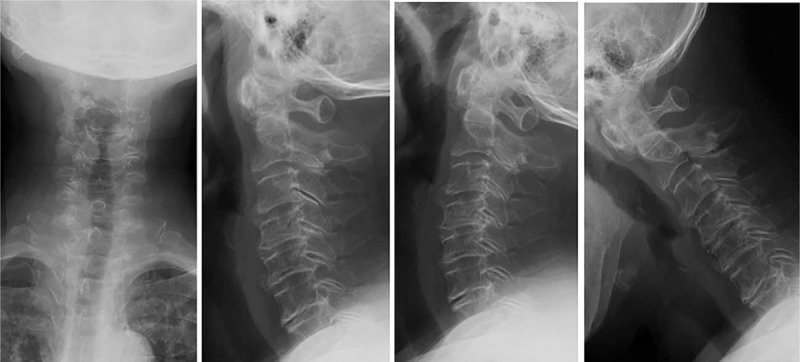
Plain cervical x-ray showed mild degenerative change without significant atlantoaxial instability.
